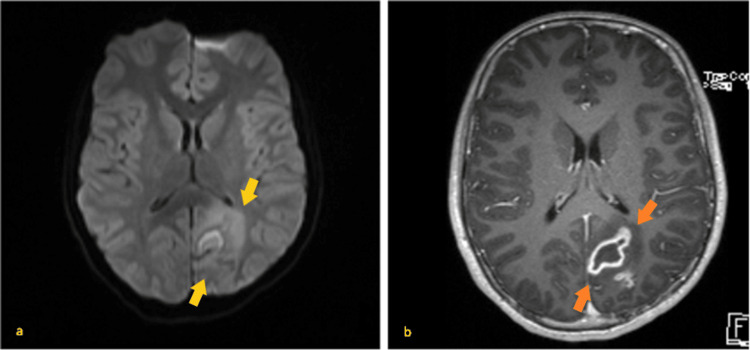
Brain MRI (a) Brain MRI showing a brain mass in the left parieto-occipital paramedian area, with mass effect, vasogenic edema, and restriction to diffusion (yellow arrows). (b) On T1-weighted, contrast-enhanced imaging, peripheral hyperenhancement can be noted (orange arrows).
